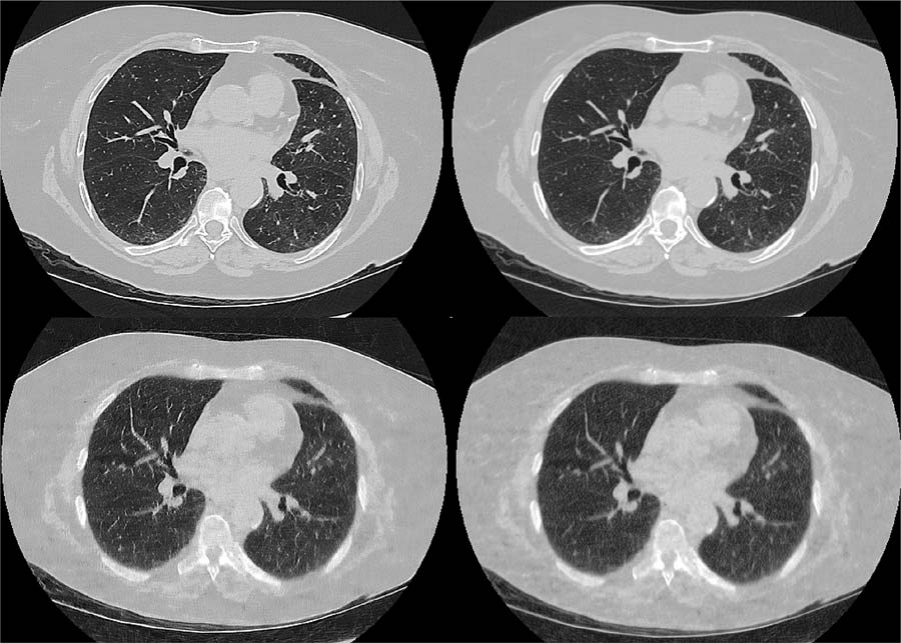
Axial CT images from the same patient acquired under full-dose (top row) and ultra-low-dose (bottom row) protocols, reconstructed using two reconstruction kernels: DLIR-H Lung kernel (left) and DLIR-H Standard kernel (right).
